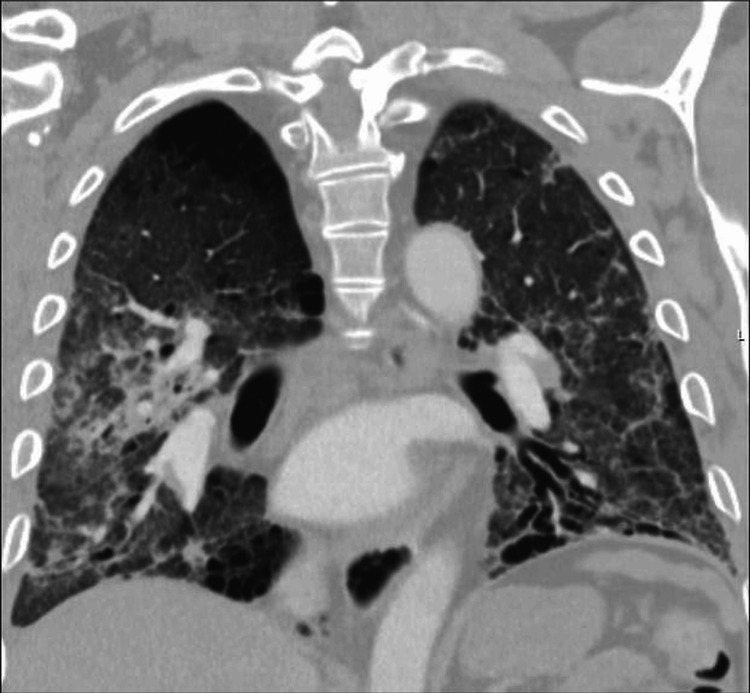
Coronal chest CT. Coronal CT view showing diffuse fibrotic changes with lower lobe predominance, volume loss, and architectural distortion. The main pulmonary artery appears dilated, raising concern for pulmonary hypertension associated with progressive fibrotic interstitial lung disease.
